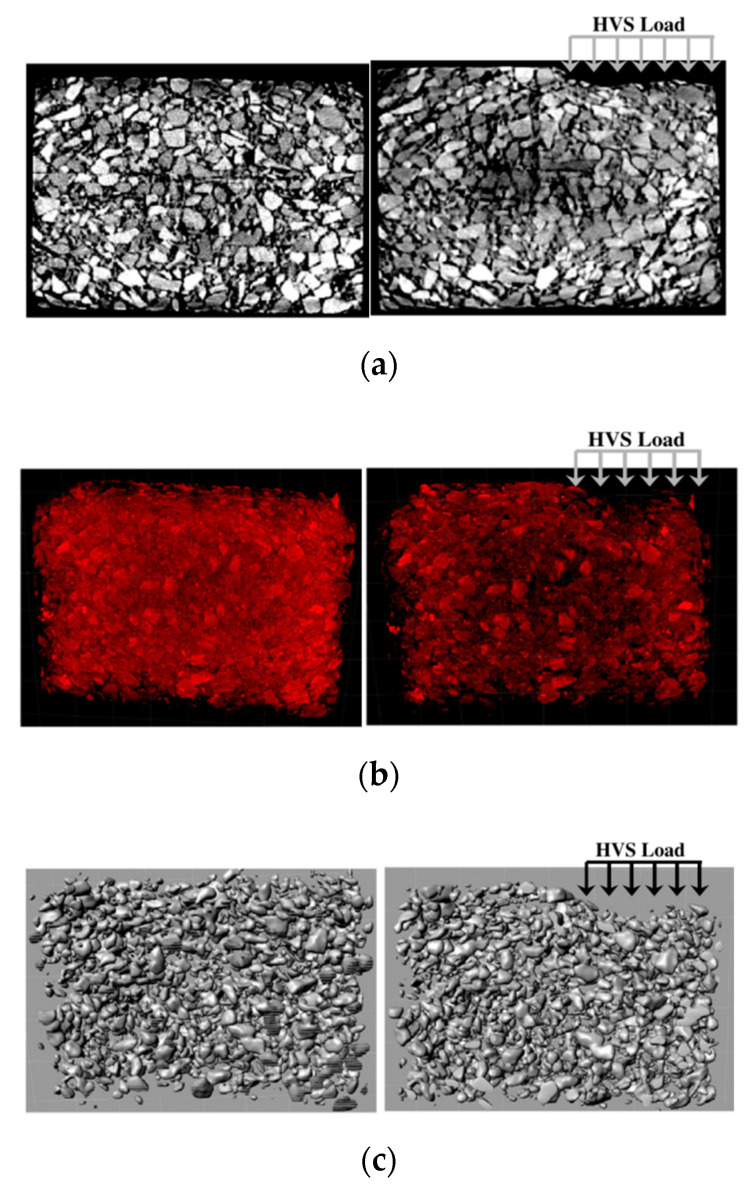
The procedure followed for aggregate domain creation: (a) unprocessed 2D X-ray CT image used for the development of image stacks (left: before HVS trafficking and right: after HVS trafficking), (b) 3D images developed from X-ray CT image stacks (left: before HVS trafficking and right: after HVS trafficking), and (c) 3D aggregate volumes (left: before HVS trafficking and right: after HVS trafficking). Note: direction of HVS traffic is out of the page. Reprinted with permission from [88]. 2012, Construction and Building Materials, Elsevier.
